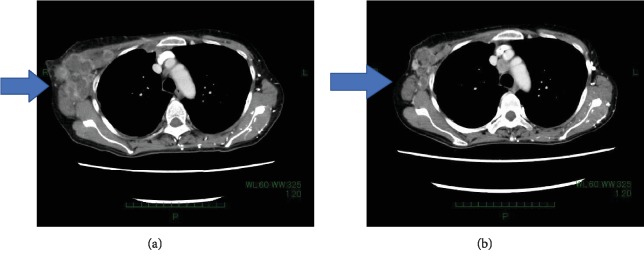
(a) CT scan section showing right axillary lymph nodes before treatment. (b) CT scan section showing right axillary lymph nodes 6 weeks after treatment initiation. Arrow shows right axillary lymph nodes being decreased after treatment.
